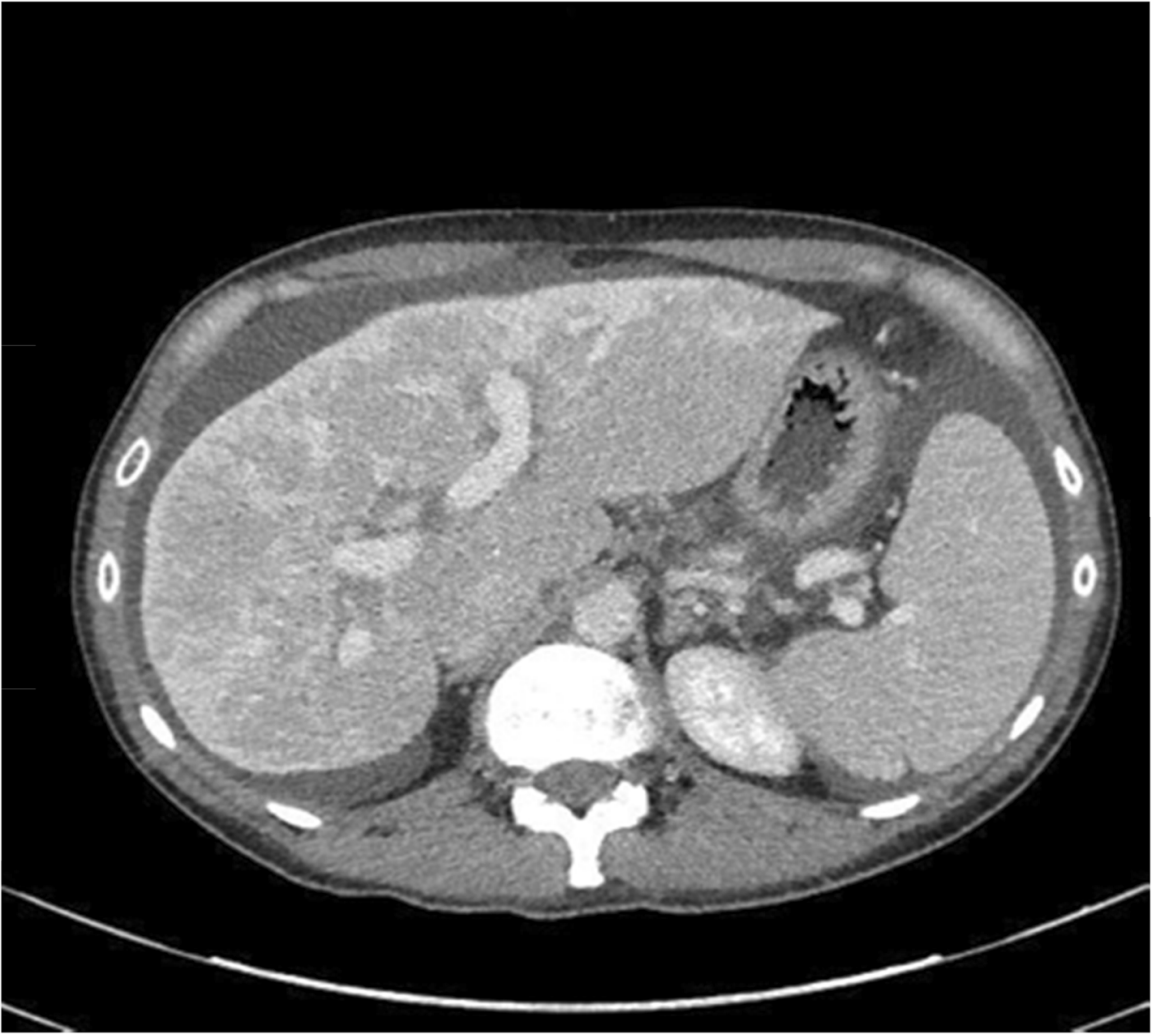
Computed Tomography scan showing unusual parenchymal enhancement with a “flip flop” pattern of reduced enhancement of the peripheral liver in the arterial phase and retention in the portal venous phase
